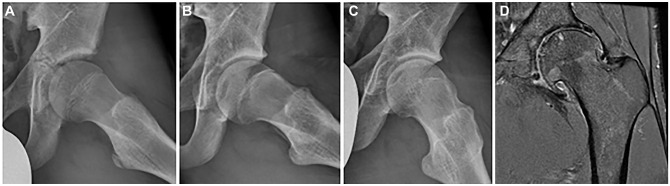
Example of a participant with 3 consecutive frog-leg lateral radiographs of the left hip at (A) baseline (aged 14 years), (B) 2.5-year follow-up (aged 16 years), and (C) 5-year follow-up (aged 19 years) showing the development of cam morphology over time. (D) Coronal proton density–weighted fat-suppressed magnetic resonance imaging of the same left hip at 12-year follow-up (aged 26 years) showing severe and diffuse acetabular and femoral cartilage loss, a subchondral cyst in the femoral head, and osteophytes.
